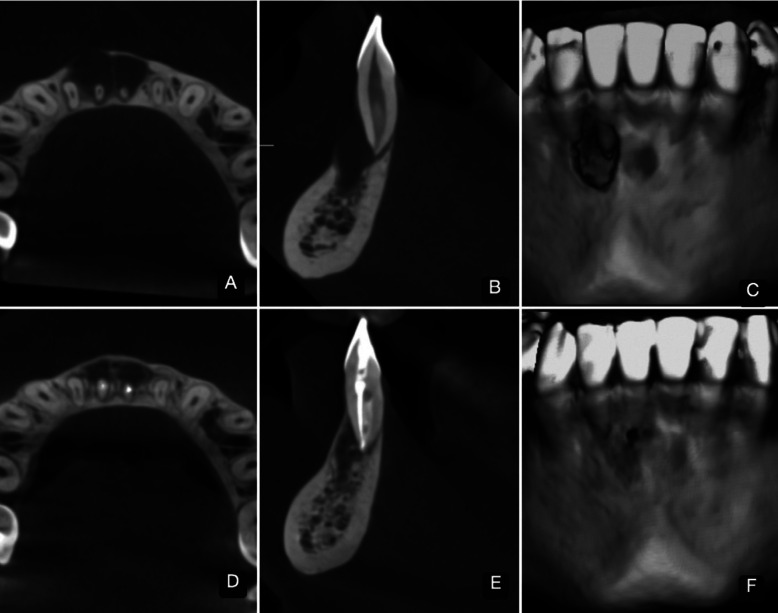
CBCT images of Clinical Case #2. A-B-C; Axial and sagittal view and three-dimensional reconstruction of the pre-treatment state. D-E-F; Axial and sagittal view and three-dimensional reconstruction of the post-treatment state after 6 months. A bony partition can be seen between the two hypodense focuses, with peripheral areas of bone neoformation and almost complete closure of the vestibular fenestration.
